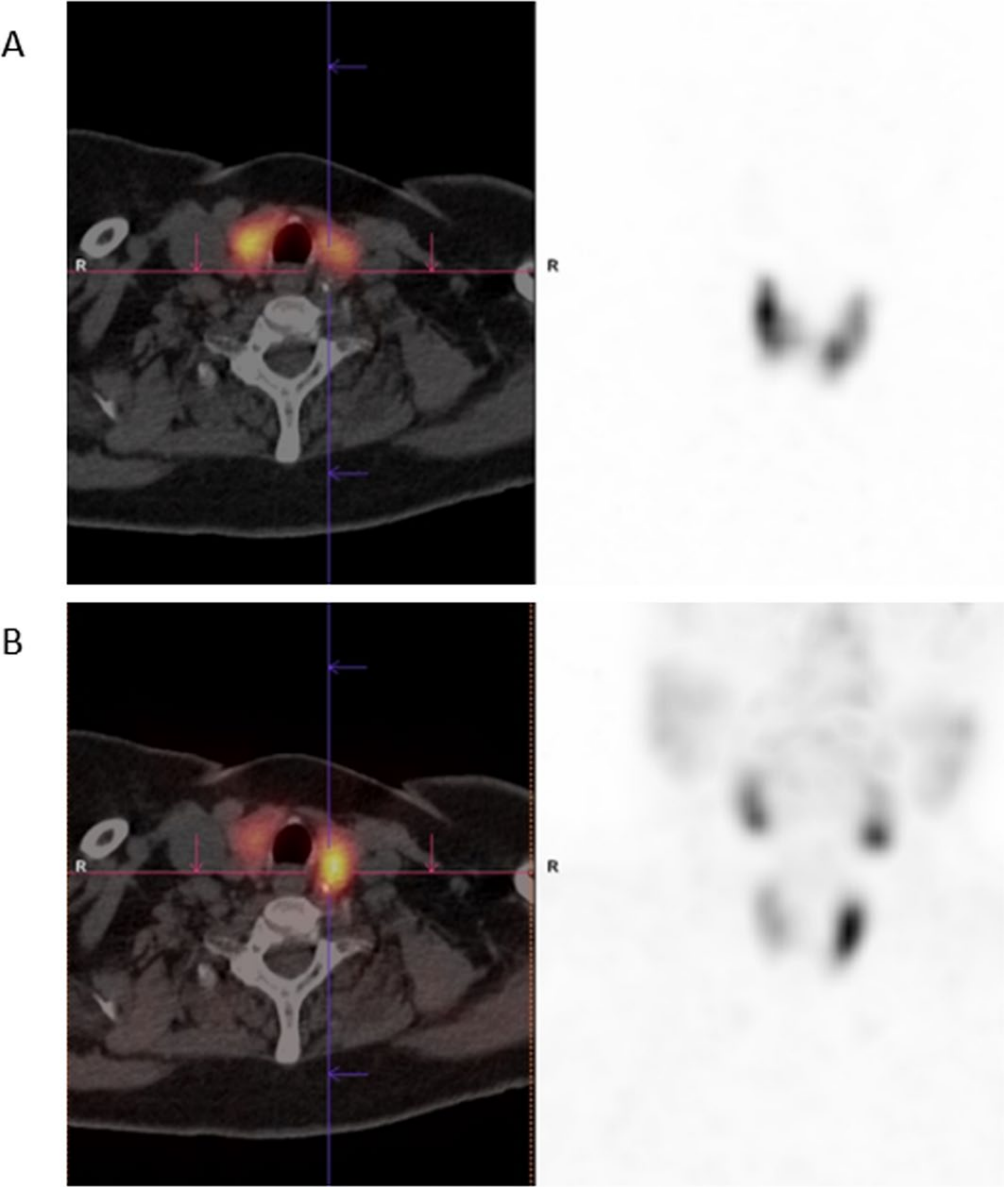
A dual isotope ^123^I/^99m^Tc-sestamibi SPECT/CT confirmed the presence of a ^99m^Tc-sestamibi-avid nodule (lower row; B, without ^123^I-uptake upper row; A) posterior from the left thyroid lobe
